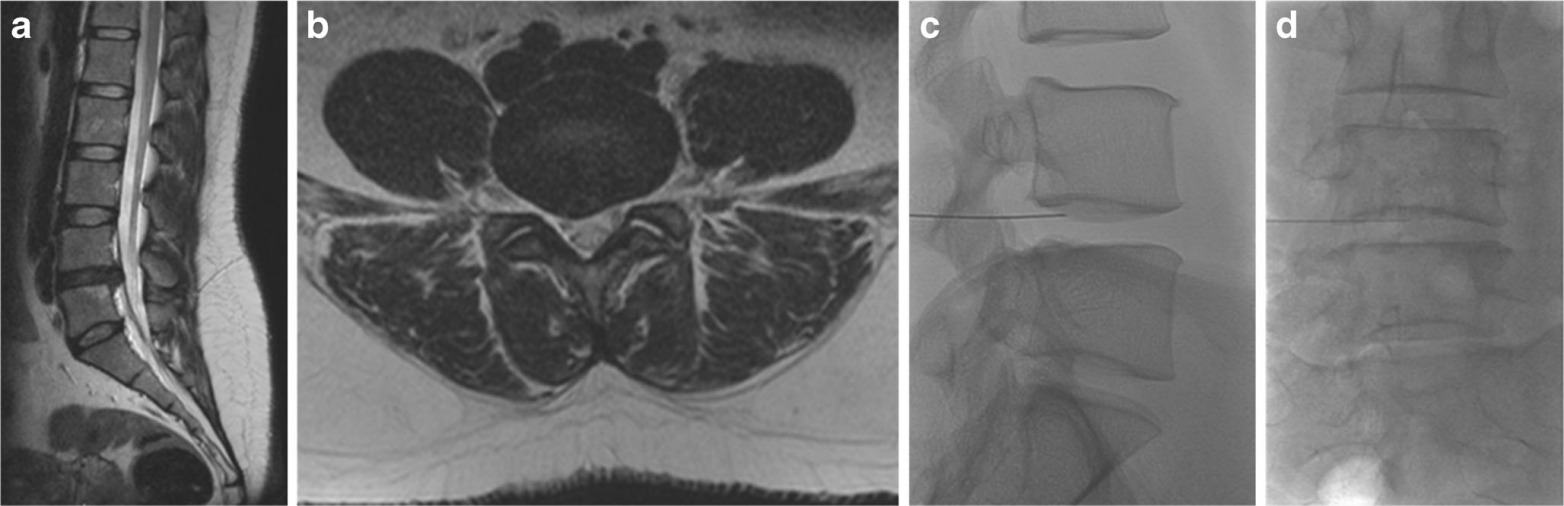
A 34-year-old women complaining of right lumbosciatica of 2-year duration and treated with ozone chemonucleolysis. a MRI T2-weighted sagittal image showing an extruded disc herniation at the level of L4–5, with pressure on the dural sac. b The corresponding axial MR T2-weighted image shows the disc herniation located at a right para-median site, compressing the dural sac as well as the traversing L5 nerve roots. c, d Lateral and anteroposterior fluoroscopic images showing the needle inside the disc center
